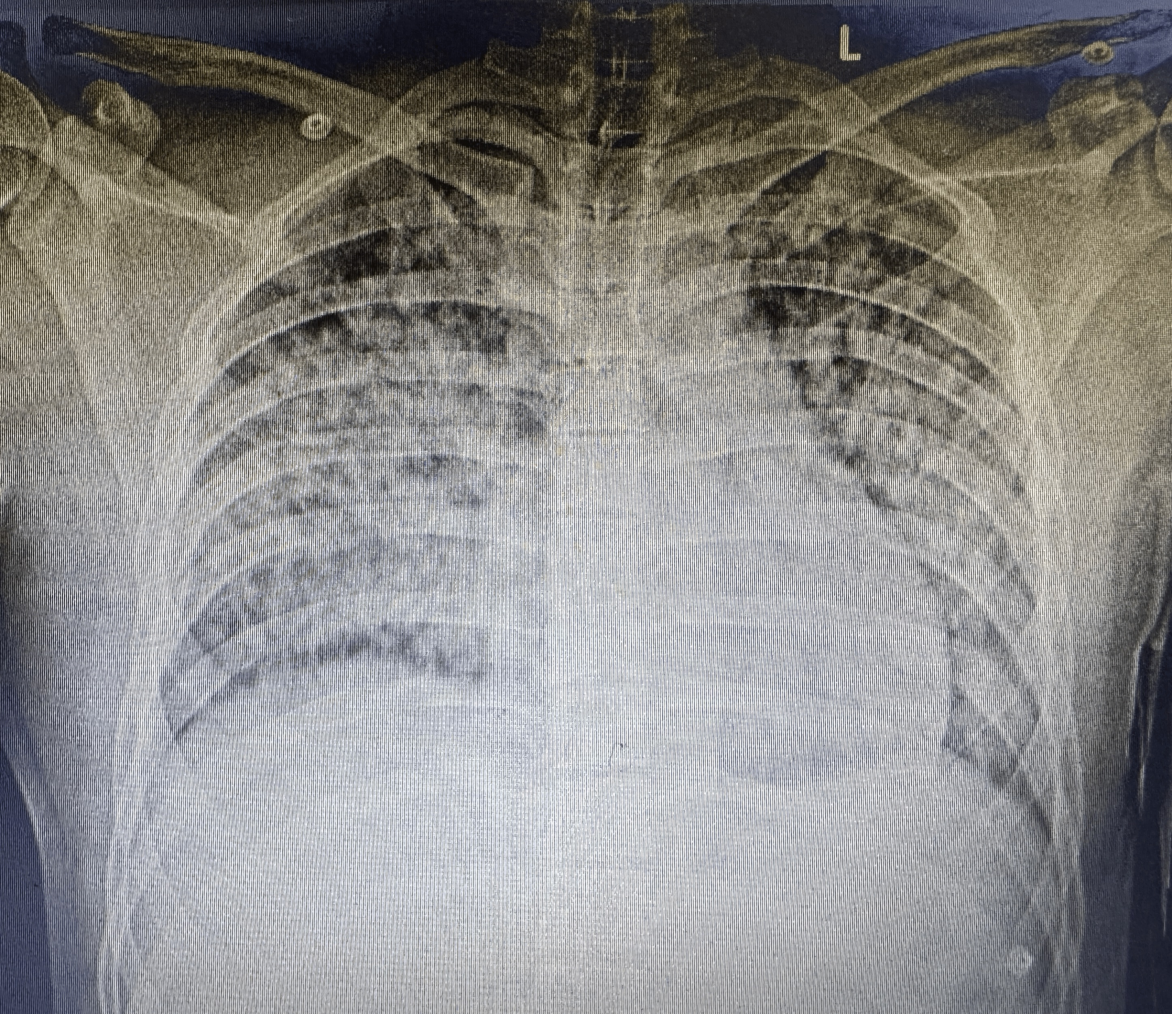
Chest X-ray showing bilateral diffuse non-homogenous fluffy opacities suggestive of diffuse pulmonary hemorrhage.
